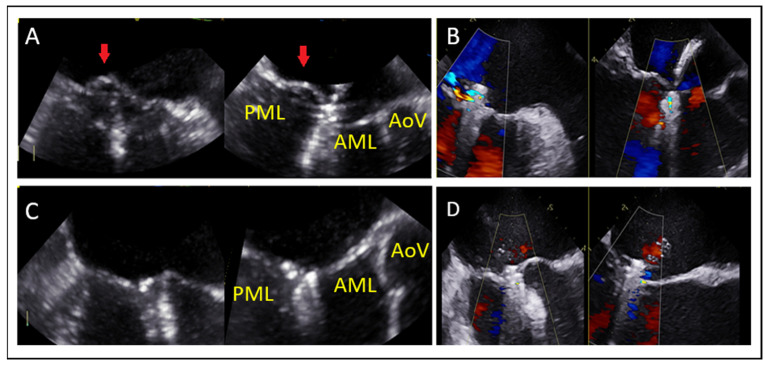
Residual leaflet prolapse after the deployment of the first device and subsequent correction with an additional device implanted medially to the first. (A) A 2D echocardiographic view demonstrates persistent leaflet prolapse, medially to the initial device. (B) The corresponding color Doppler image reveals a regurgitant jet at the medial aspect, indicating residual mild MR. (C) A 2D echocardiographic view, similar to (A), shows no residual prolapse after the placement of a second device, confirming optimal anatomical correction. (D) The corresponding color Doppler image, similar to (B), demonstrates non-significant residual MR. AML: anterior mitral leaflet, PML: posterior mitral leaflet, AoV: aortic valve.
